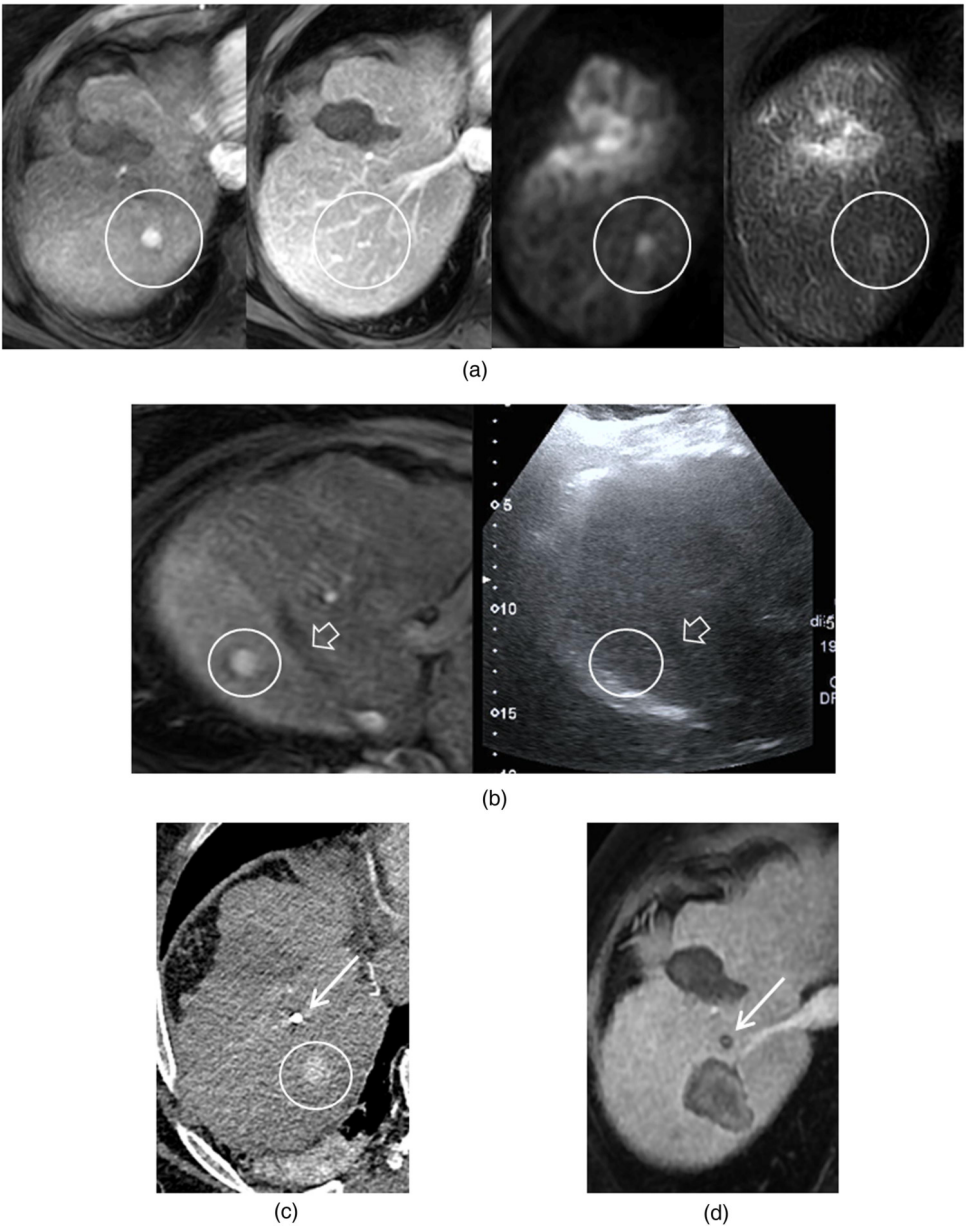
Small recurrent hepatocellular carcinoma occurring 6 months after initial thermal ablation of a first lesion. Lesion detection: (a) A 10 mm nodule is identified adjacent to the ablation scar on T1 arterial-phase MRI, T2, and diffusion-weighted (b1000) imaging. It is not visible on portal-phase imaging. Lesion localization: (b) Fusion imaging with arterial-phase MRI did not allow confident visualization of the nodule. A fiducial marker was therefore placed under ultrasound guidance, posterior to the right hepatic vein (hollow arrow), in the region corresponding to the tumor on MRI. Due to poor echogenicity, the marker was not visualized on ultrasound after withdrawal of the applicator needle. (c) Immediate post-procedural CT showed the marker (white arrow) positioned approximately 4 cm anterior to the tumor. Despite this suboptimal placement, ablation could be performed under CT guidance by targeting posterior to the marker. (d) One-month follow-up MRI demonstrated an ablation zone encompassing the tumor, which was no longer visible.
